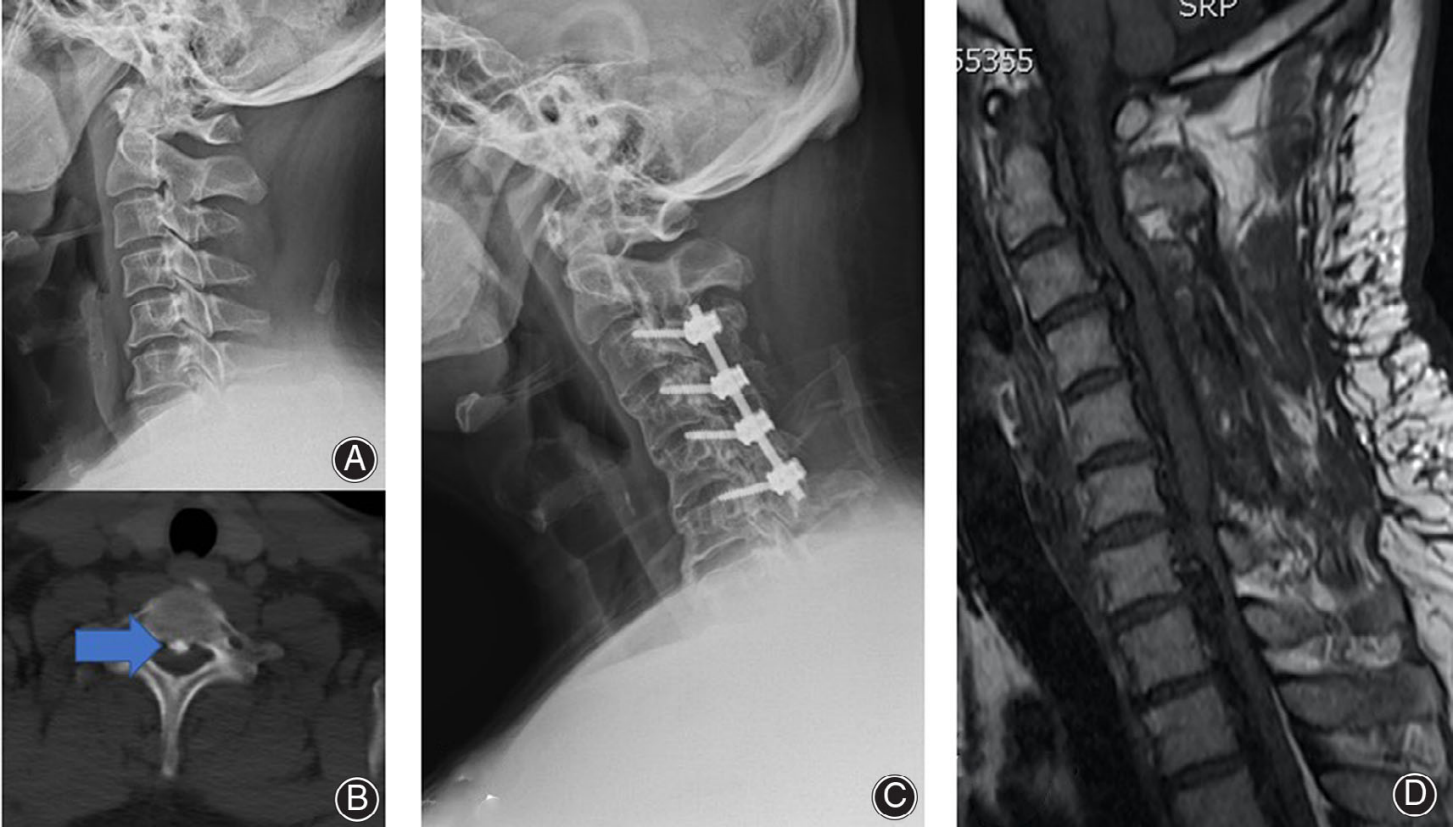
OPLL in a 34‐year‐old male with neurological symptoms. (A, B) Preoperative radiographic images. The blue arrows indicate ossification of the posterior longitudinal ligament in the C7 segment. (C) Postoperative X‐ray images showed pedicle screw position was accurate. (D) Postoperative MRI showed that spinal cord decompression was complete.
